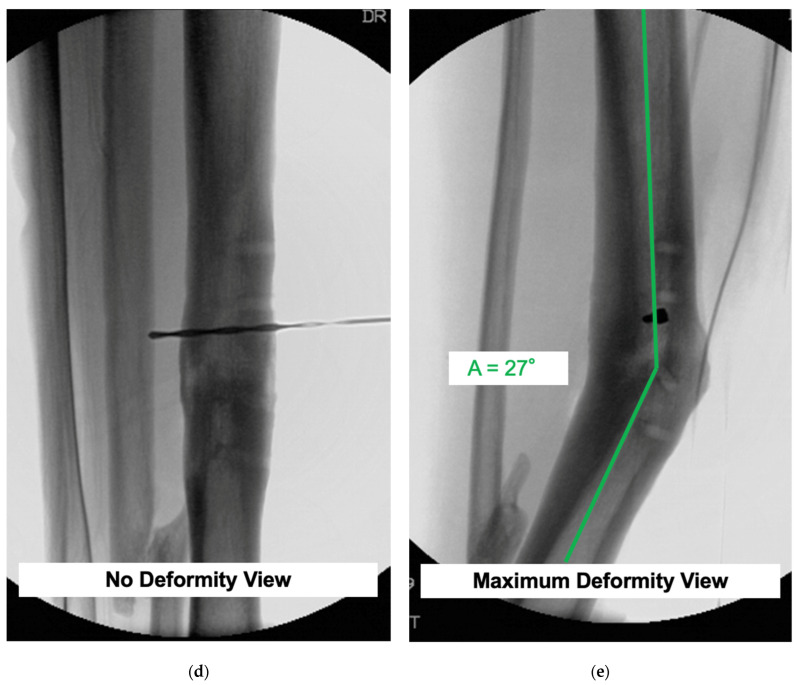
See Case 1 description for details. (a–e) demonstrate how to calculate the true angular deformity (A) and the orientation angle (α) from coronal and sagittal plane radiographs.
